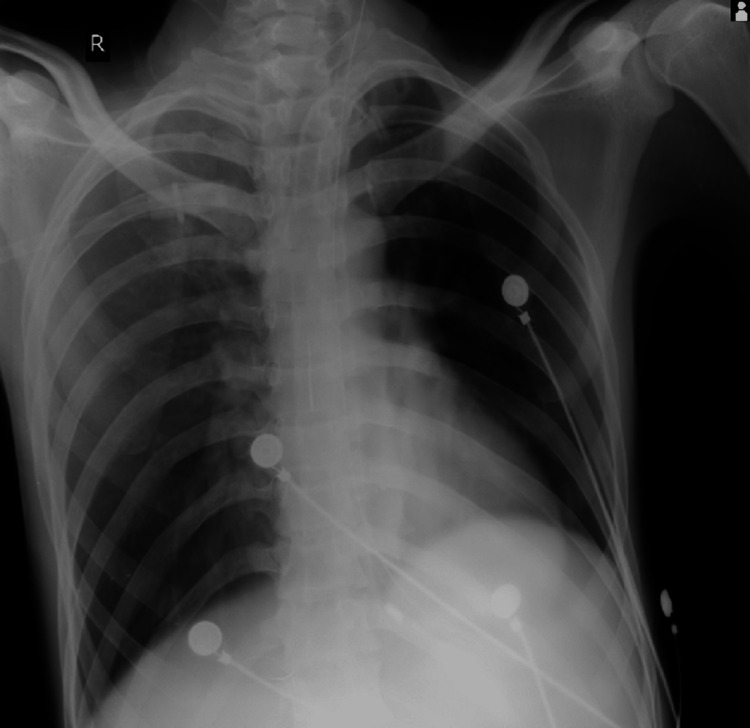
X-ray of January 5, 2023, showing patch in middle lobe with the possibility of ventilator-associated pneumonia
